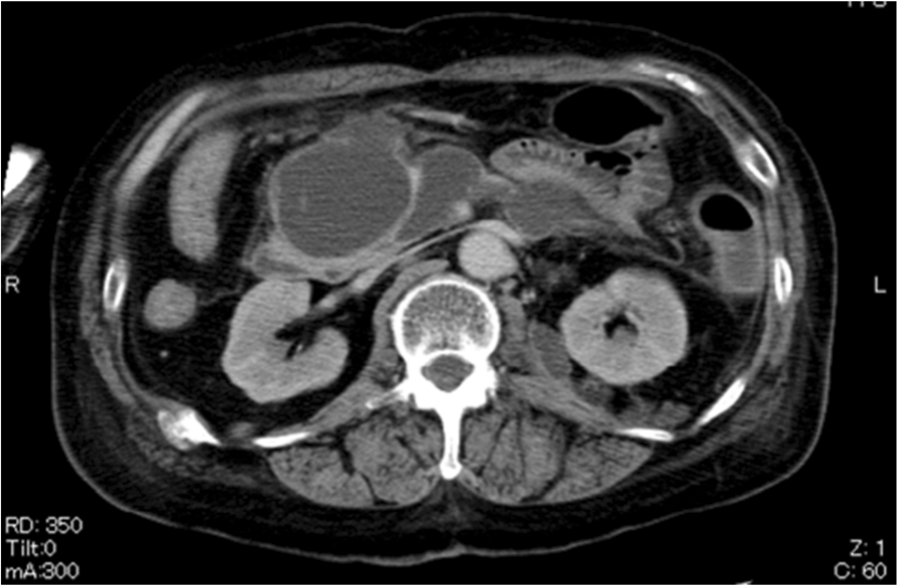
Abdominal CT shows swelling of the pancreas head, multiple pseudopancreatic cysts, and also atrophic kidneys.
